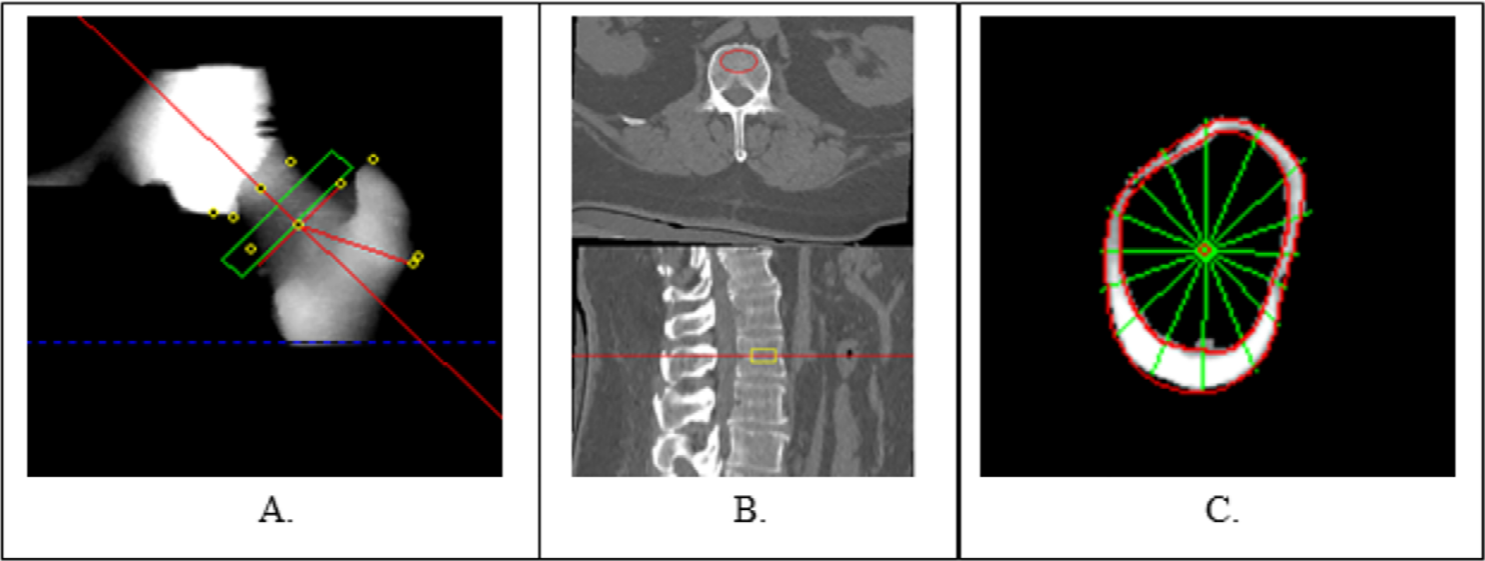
(*A*) Exemplar hip volumetric BMD analysis using computed tomography X‐ray absorptiometry hip module. (*B*) Lumbar spine analysis of volumetric BMD using three‐dimensional spine module. (*C*) Cortical thickness analysis of femoral neck using the Bone Investigational Toolkit software (Mindways Software, Austin, TX, USA).
